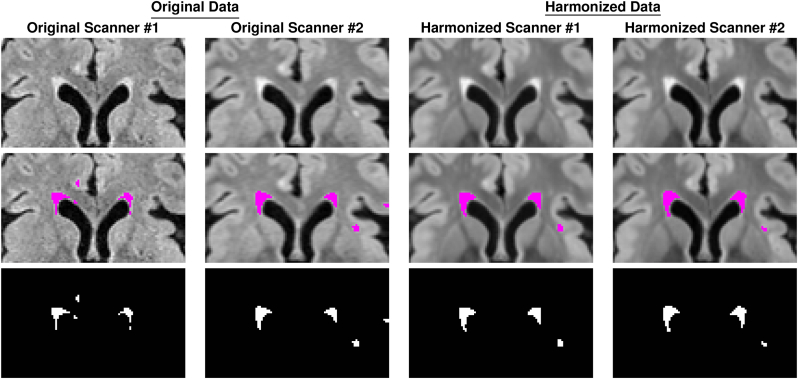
Shown in the top row is a zoomed portion of the FLAIR data from the Original Scanner #1, Original Scanner #2, Harmonized Scanner #1, and Harmonized Scanner #2. The bottom row is the corresponding manual delineations for the images in the top row. The center row is the FLAIR images (top row) overlaid with the manual masks (bottom row) colored in magenta.
